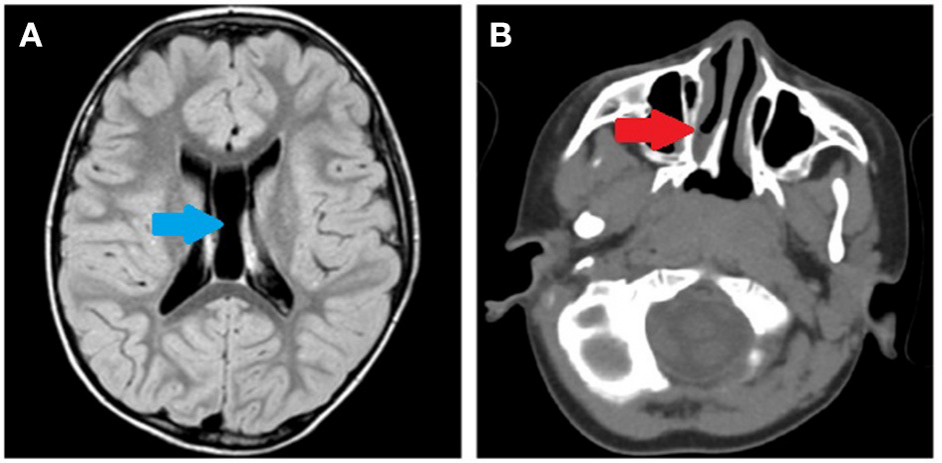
(A) Brain MRI performed at 6 years of age. Cysts of the septum pellucidum and the cavum vergae (blue arrow). (B) Brain CT scan performed at 3 years of age. Right choanal atresia (red arrow).
